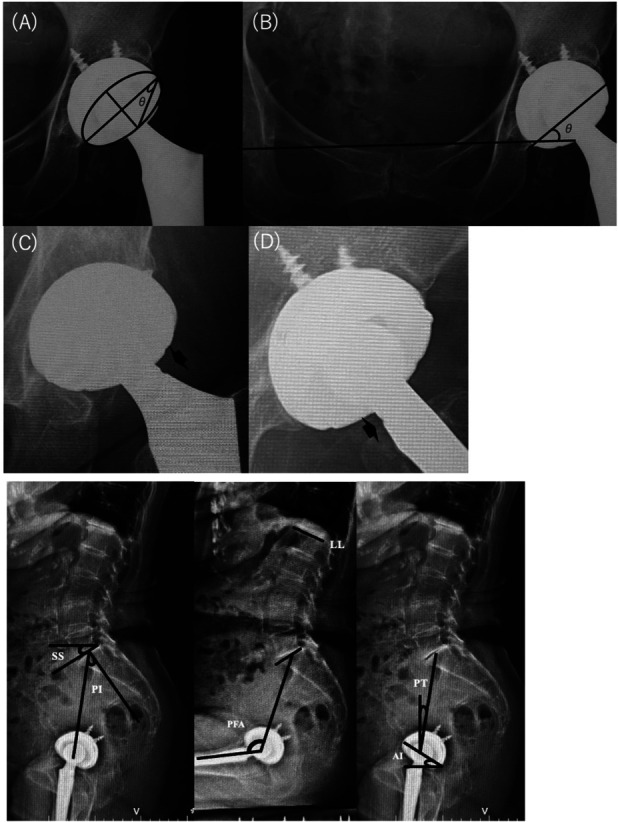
Radiographic evaluation of cup positioning and femoral neck notching (FNN) and sagittal EOS image showing the spinopelvic parameters. (Fig. 1-A) Anteversion, (Fig. 1-B) inclination, and (Fig. 1-C) lateral FNN is defined as a notch on the lateral aspect of the femoral stem neck in the anteroposterior radiographic view (closed arrow). (Fig. 1-D) Posterior FNN is defined as a notch on the lesser trochanteric side of the stem neck in the lateral radiographic view (closed arrow).
